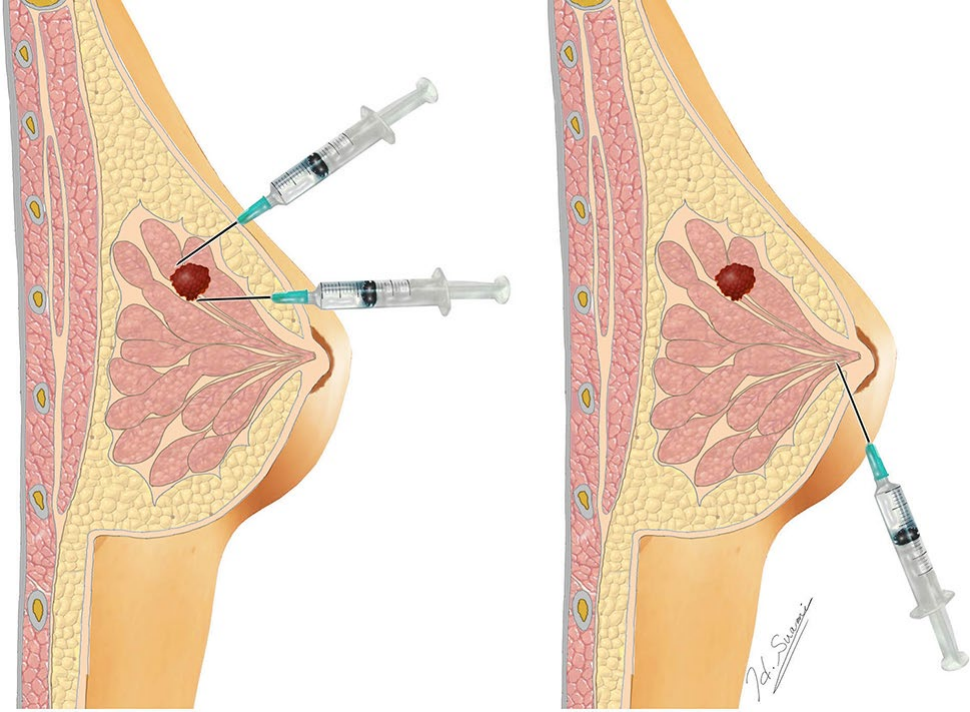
Peritumoral (PT) and subareolar (SA) injection types for administering the radiocolloid tracer during LS and SPECT/CT
